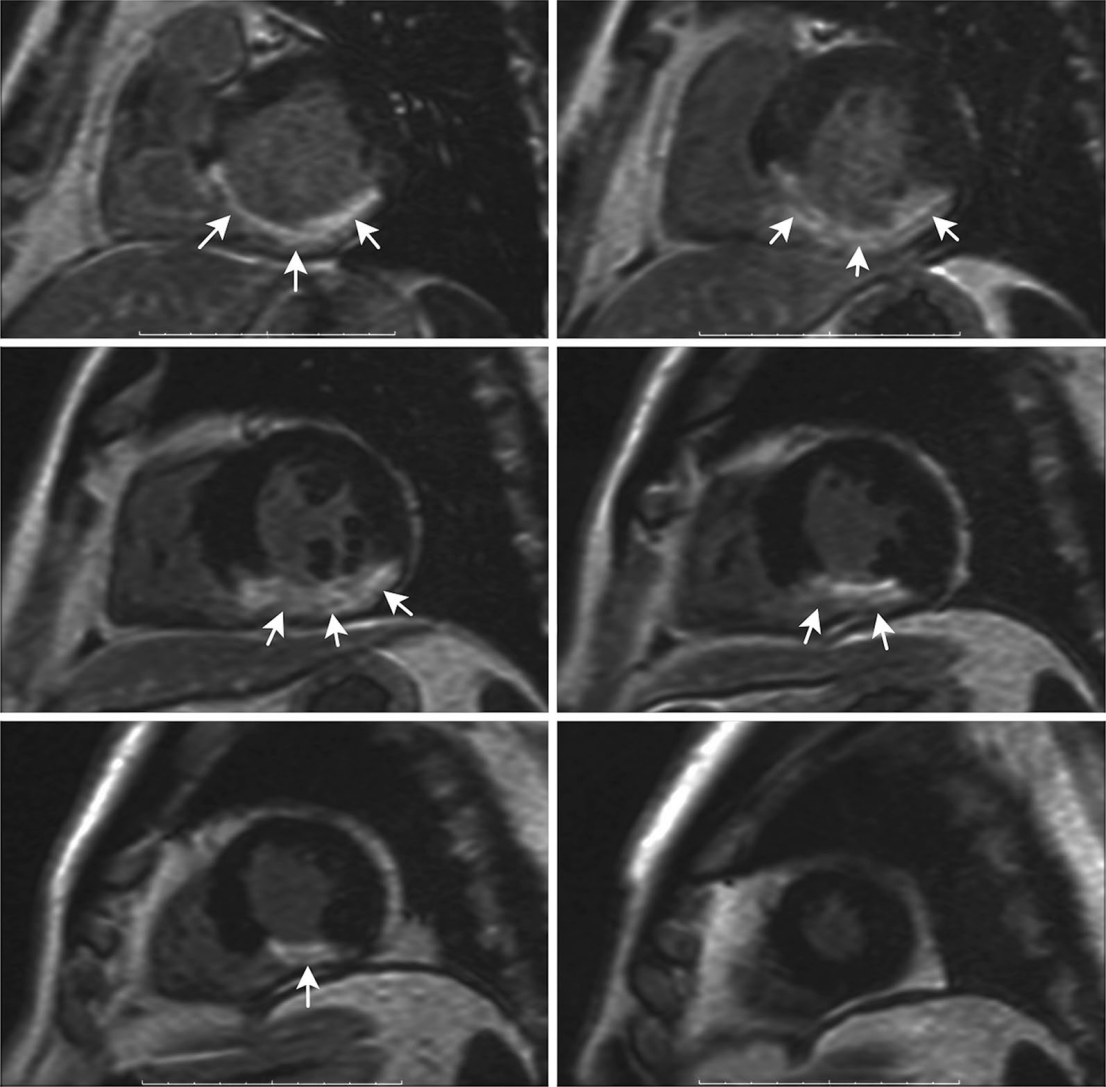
Myocardial fibrosis (white arrows) on T1-weighted images late enhancement. Short axis of the left ventricle of the heart
